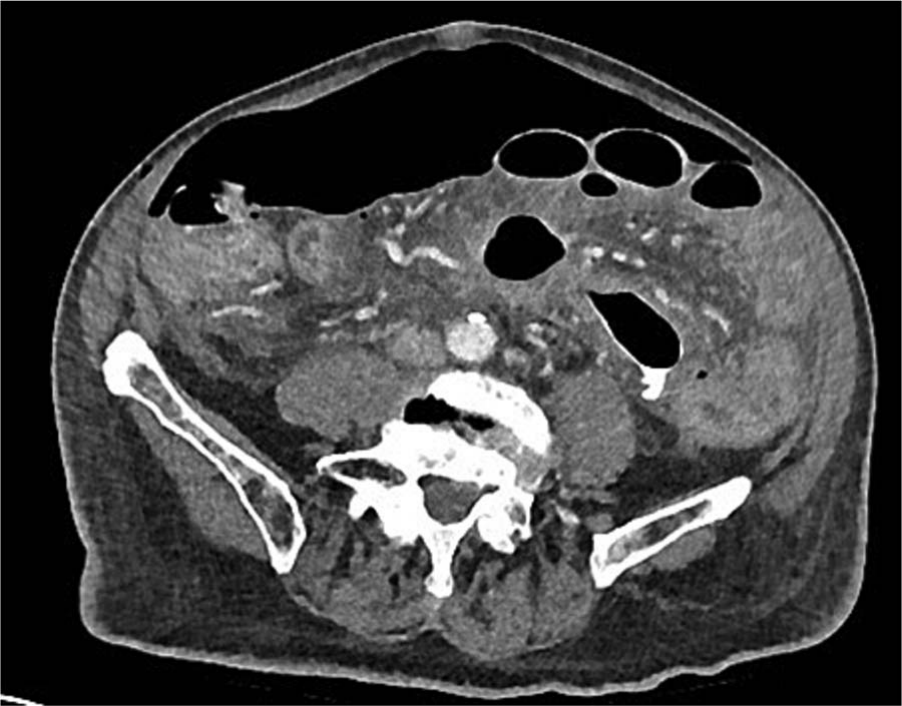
Day 10 CT scan demonstrates ileus with large volume pneumoperitoneum with free air around anastomosis.
